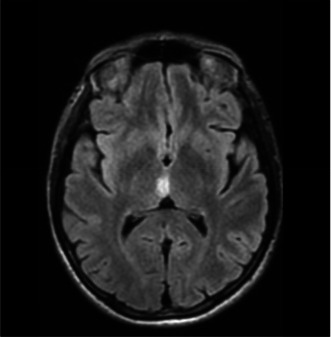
FIGURE 1 Axial T2‐FLAIR MRI showing an isolated hyperintense lesion in the right thalamus.
